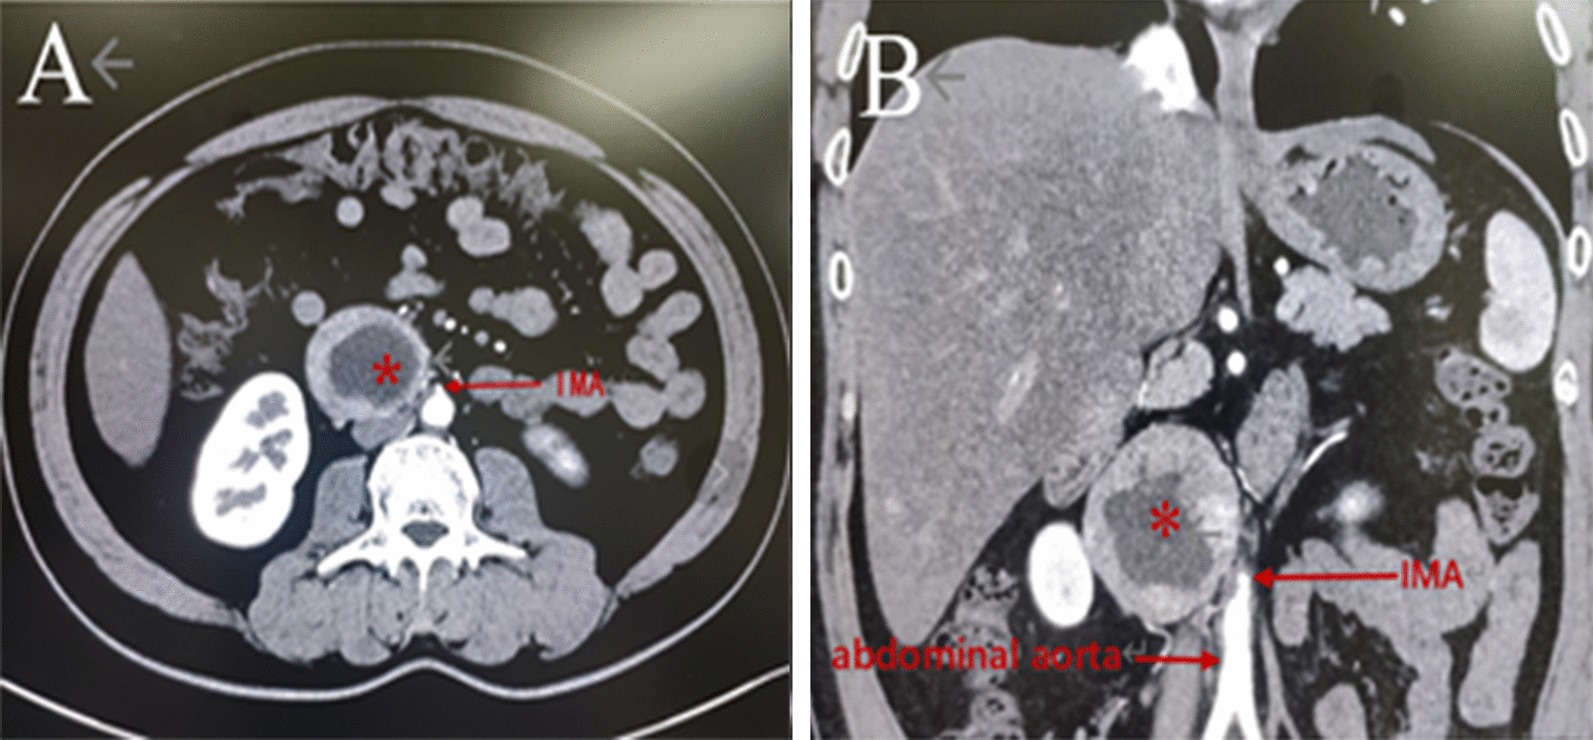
CT findings: CT shows a 7.2 × 6.5 cm right para-aortic mass (asterisk) located at the level of the inferior mesenteric artery (IMA). Transverse plane (a). Coronal plane (b)
